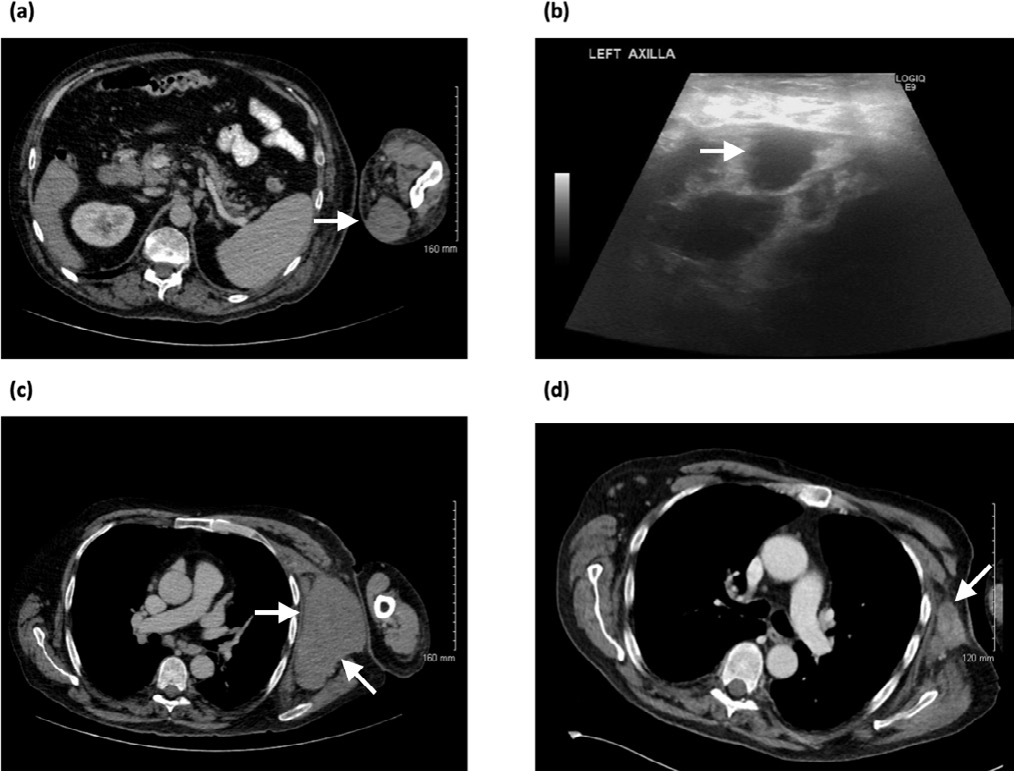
(a) Axial CT image showing a large well-defined soft tissue mass in the medial aspect of the left elbow measuring 5 × 4 cm, superficial and separate from the underlying musculature, (b) sonogram of the left axilla showing an enlarged hypoechoic pathological lymph node, (c) axial CT image showing a large conglomerate nodal mass in the left axilla, (d) end-of-treatment axial CT image showing significant reduction in size of the axillary mass.
